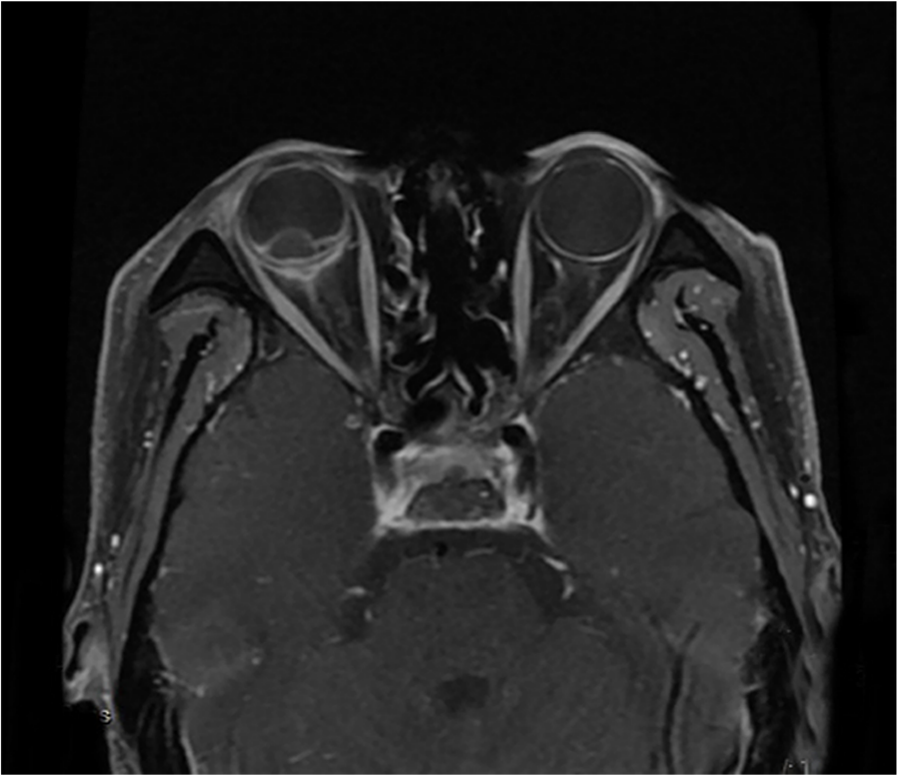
Transverse T1-weighted strengthened scanning images showed that the posterior segment of the right eye is characterized by “stratification” and irregular thickening of the posterior wall with reinforcement
